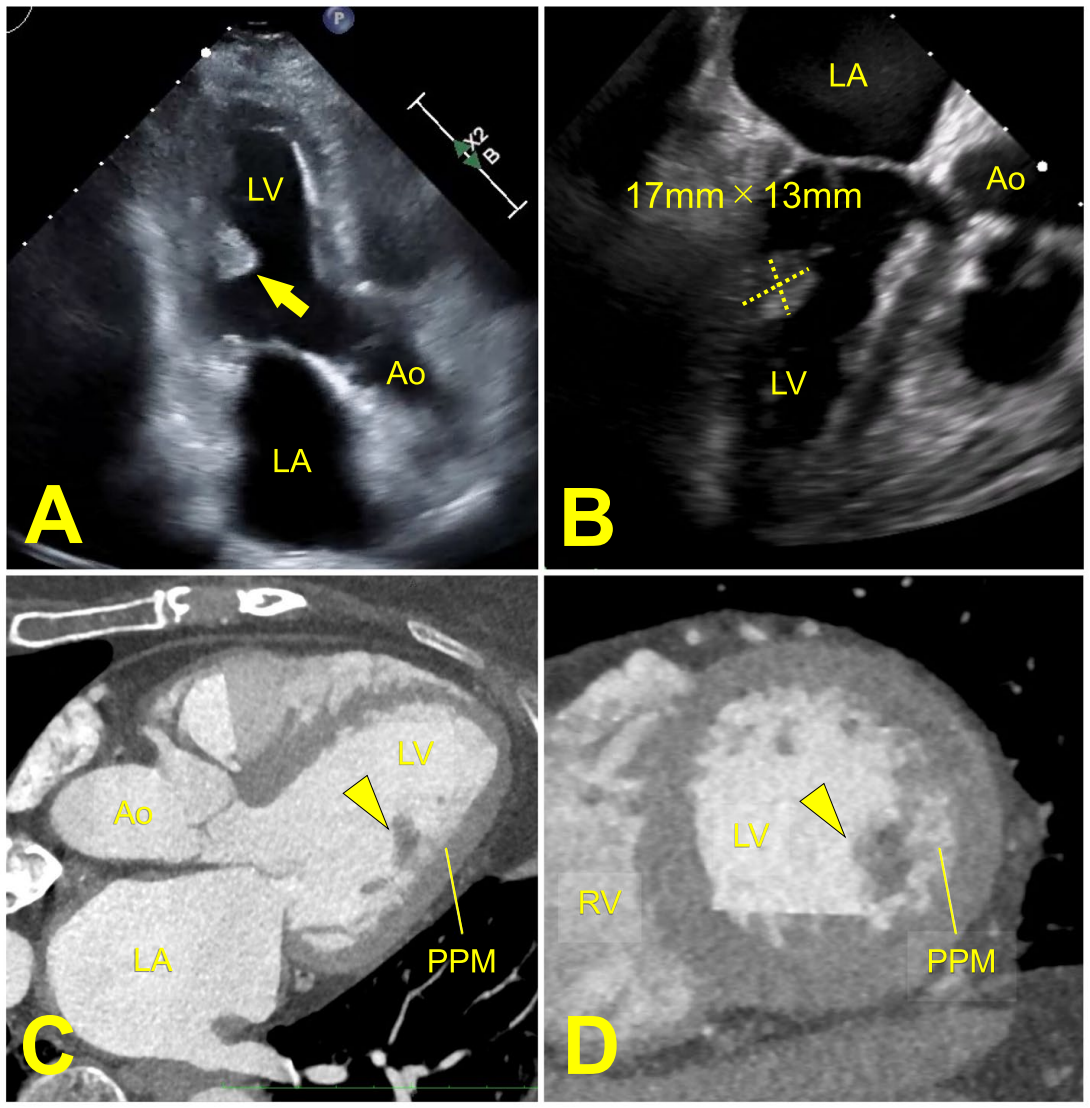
A, B Preoperative transthoracic echocardiography (A) and transesophageael echocardiography (B) reveal a highly mobile mass measuring 17 × 13 mm, attached to the posterior papillary muscle (arrow). C, D Three-lumen (C) and short-axis views (D) of cardiac computed tomography demonstrate a mass attached to the posterior papillary muscle (arrowhead). LV: left ventricle; LA: left atrium; Ao: aorta; RV: right ventricle; PPM: posterior papillary muscle
